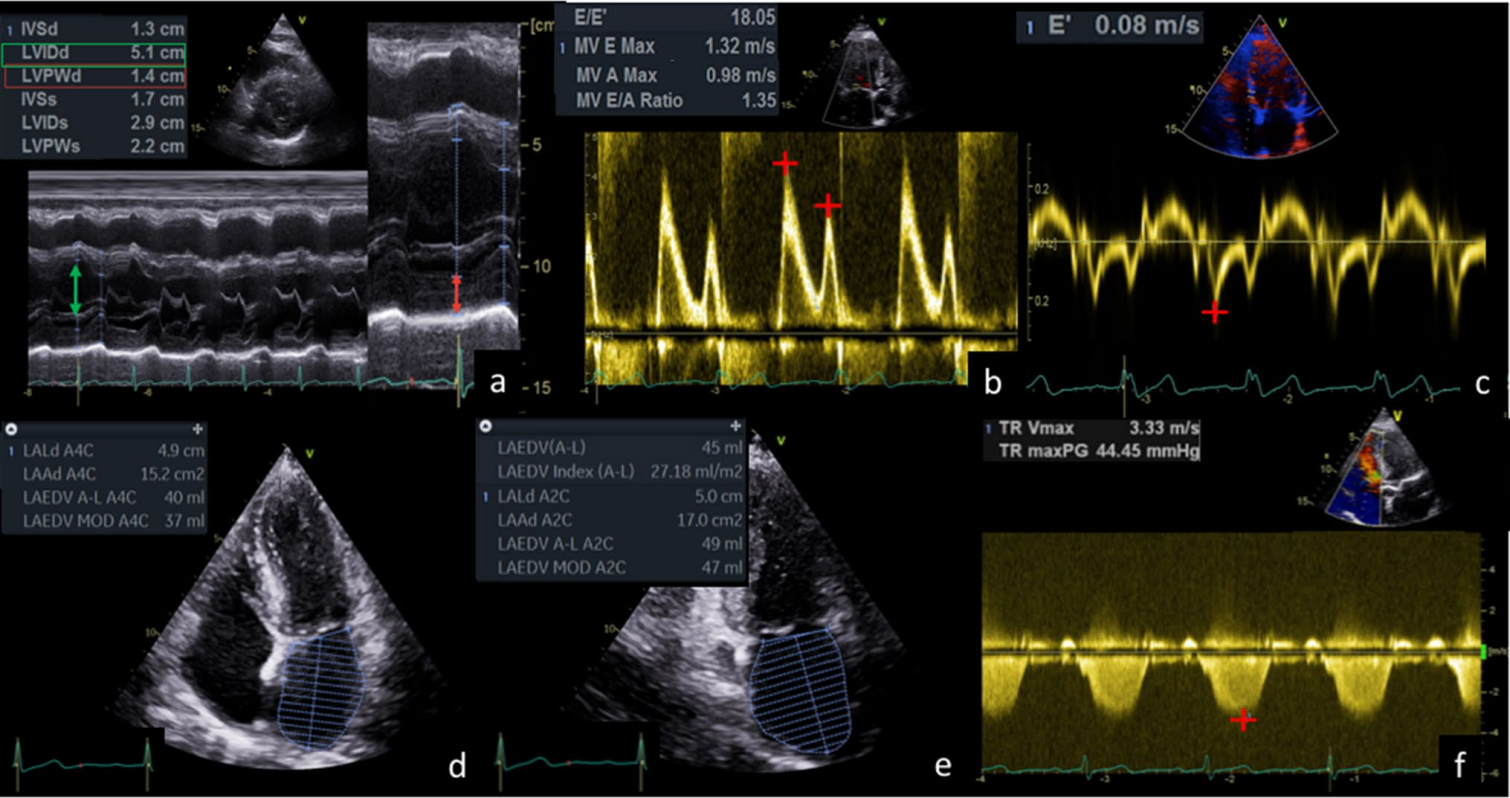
Determination of left ventricular hypertrophy (a), diastolic dysfunction (b-f) and pulmonary arterial hypertension (f) by echocardiography. IVSd = diameter of interventricular septum, LVIDd = left ventricular internal dimension at end-diastole, LVPWd = left ventricular posterior wall diameter, LAEDV = left atrial end diastolic volume, TR = tricuspid regurgitation, Vmax = maximum velocity, maxPG = maximum pressure gradient, MV = mitral valve
